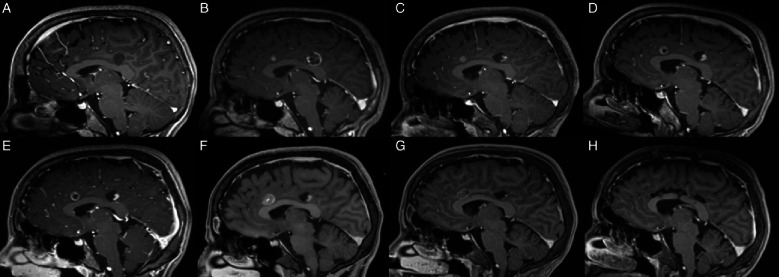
Serial T1-contrast-enhanced sagittal MR images of case #3. A At diagnosis, showing the posterior cingulate larger lesion, and a small anterior cingulate enhancing lesion. B At the end of the first LITT, the enhancement encompasses the entire posterior lesion. C–E On follow-up, the anterior cingulate lesion continued to grow. F At the end of the second LITT showing good ablation of the anterior cingulate lesion. G Six months after the second LITT. H After open resection of the cingulate gyrus
